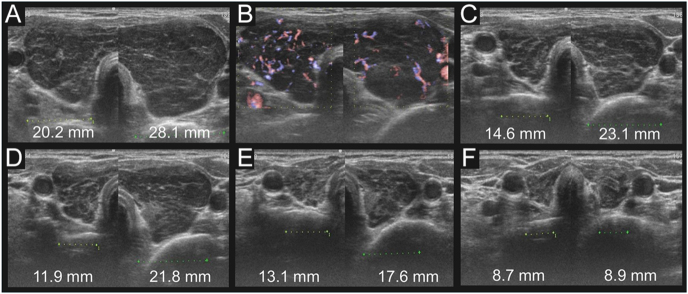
Ultrasonographic changes in the patient with thyroid MTX-LPD after MTX withdrawal. At the first visit, the thyroid showed diffuse enlargement and decreased echogenicity. (A) Color Doppler ultrasonography demonstrated diffuse, moderately increased vascularity. (B) The transverse diameter of each lobe (the numbers in the figure represent measurements in mm) was measured at the first visit and at 2-, 4-, 8-, and 12-months. (A, C, D, E, F) It showed significant shrinkage over time.
